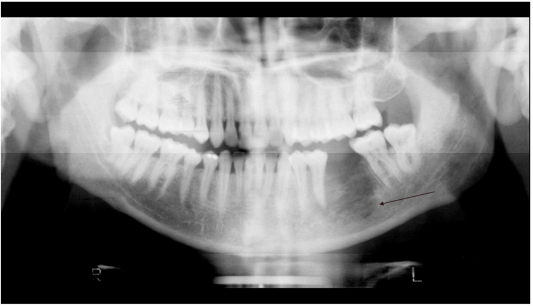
Orthopantomogram shows lytic destruction of the left side involving the left first molar bed (arrow) and the root of the 2nd premolar.
